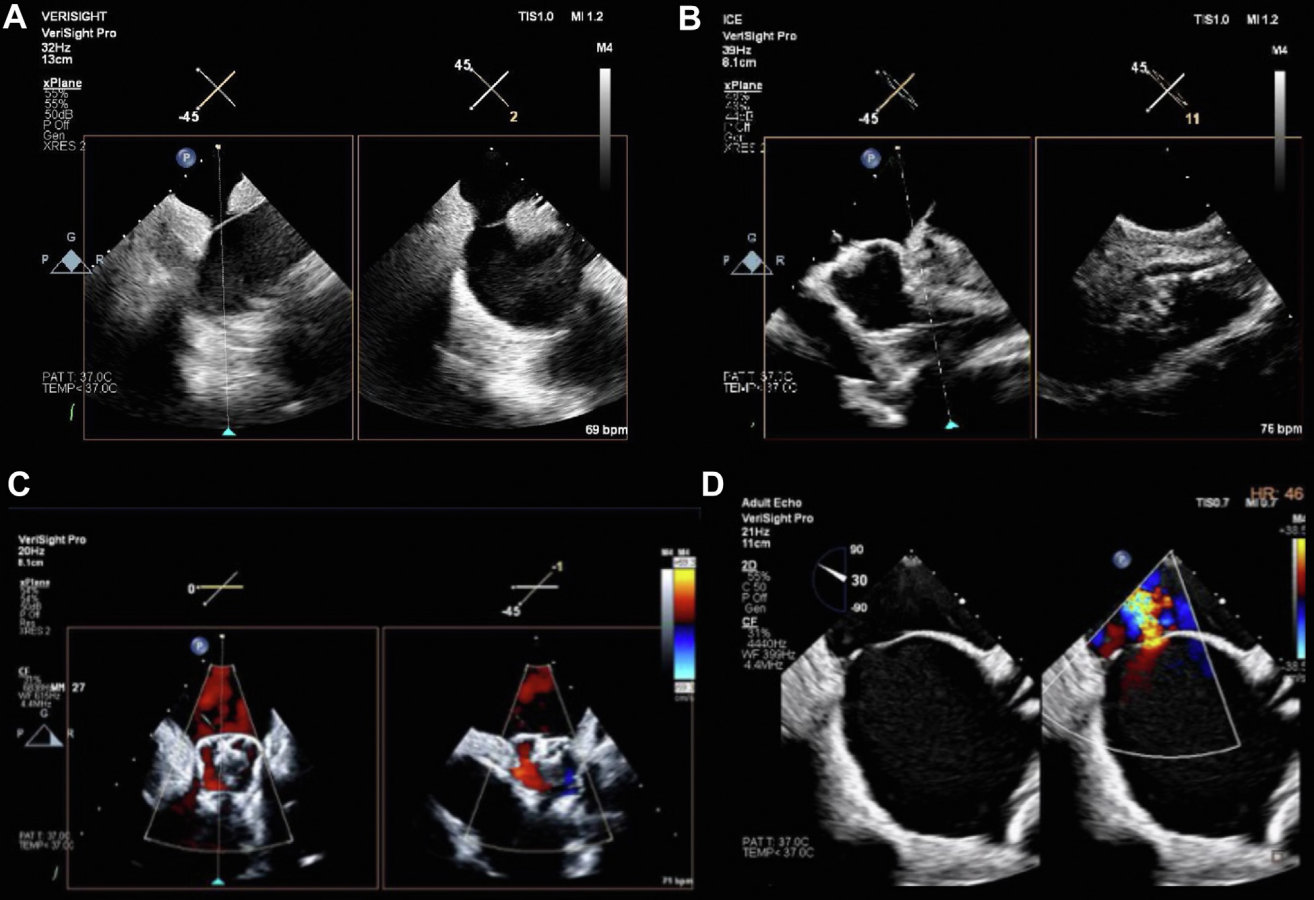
3-Dimensional Intracardiac Echo-Guided Left Atrial Appendage Occlusion (A) Transseptal puncture in the mid-mid position; (B) LAA imaging with the probe positioned within the left atrium; (C) the PASS (Position, Anchor, Sizing, Seal) criteria assessment; (D) postimplant ASD assessment on color Doppler. ASD = atrial septal defect; LAA = left atrial appendage.
